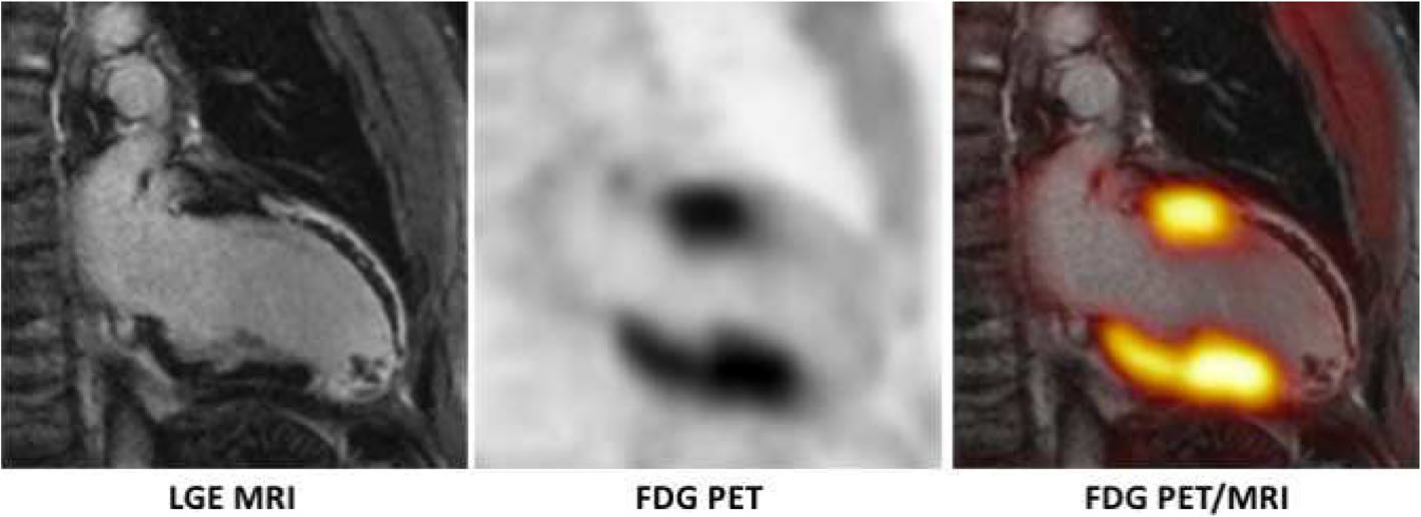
Viability assessment with hybrid FDG PET/MRI. A FDG PET/MRI (viability protocol with glucose loading) was performed in a mid-aged man early after acute ST-elevation myocardial infarction with occlusion of the left anterior descending (LAD) coronary artery. The inferior wall and the basal part of the anterior wall demonstrate no late gadolinium enhancement (LGE) and a normal FDG uptake and are therefore PET/MRI vital. The remaining parts of the heart in this two-chamber-view demonstrate transmural LGE with subendocardial microvascular obstruction in the anterior wall and almost absent FDG uptake and are therefore judged PET/MRI non-vital
